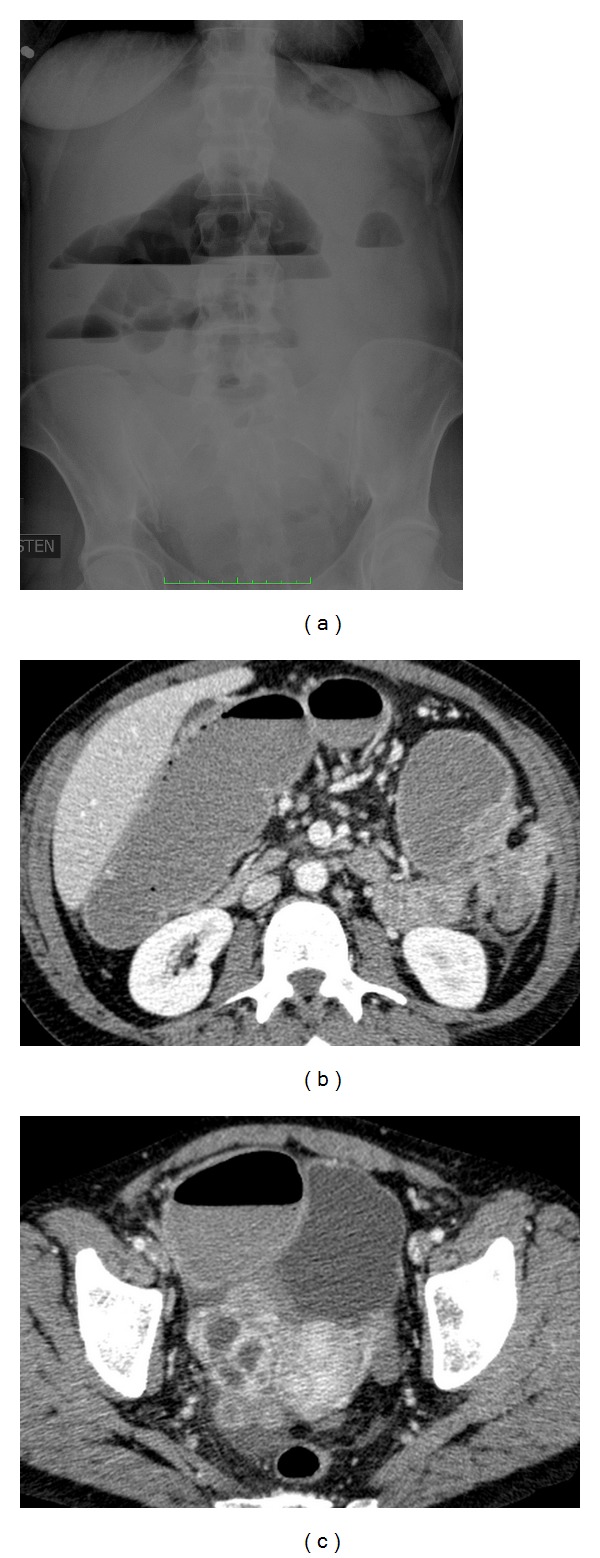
(a) An abdominal radiograph reveals multiple fluid levels and a dilated bowel segment (arrow heads), (b) a CT scan from upper abdomen exhibits dilated colon structure (black arrowheads), narrowed colonic segment (white arrowhead), and extraluminal infiltration (arrow), and (c) A CT scan from lower abdomen exhibits cystic involvement in right ovary (arrowheads) as well as enlarged colon (asterisk).
